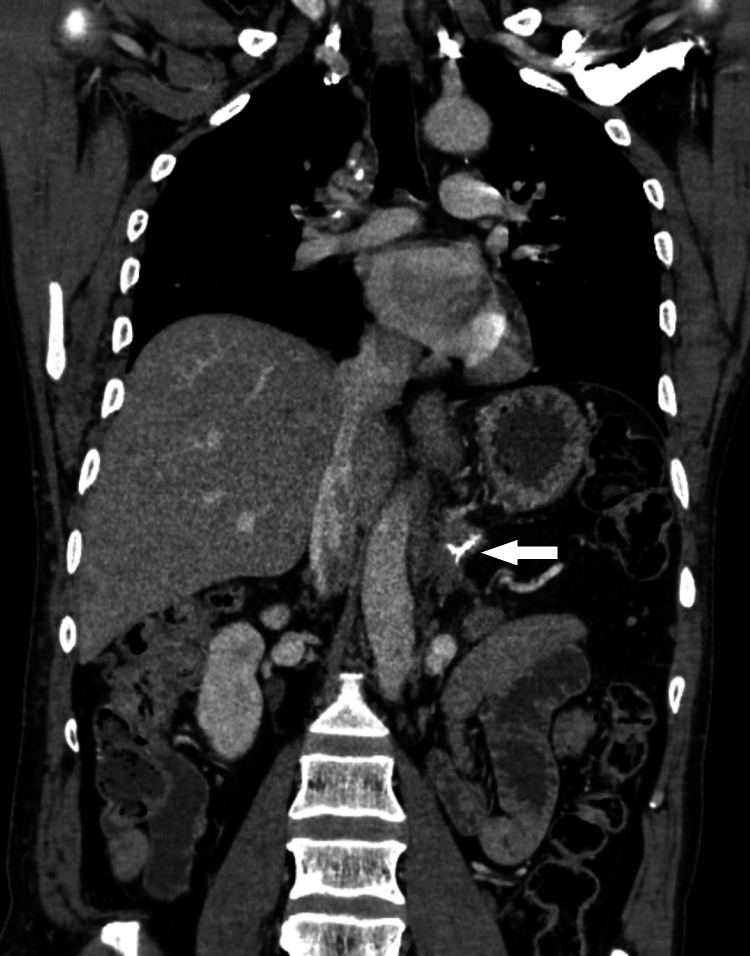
CT chest/abdomen coronal image showing post-operative changes with no evidence of recurrence
